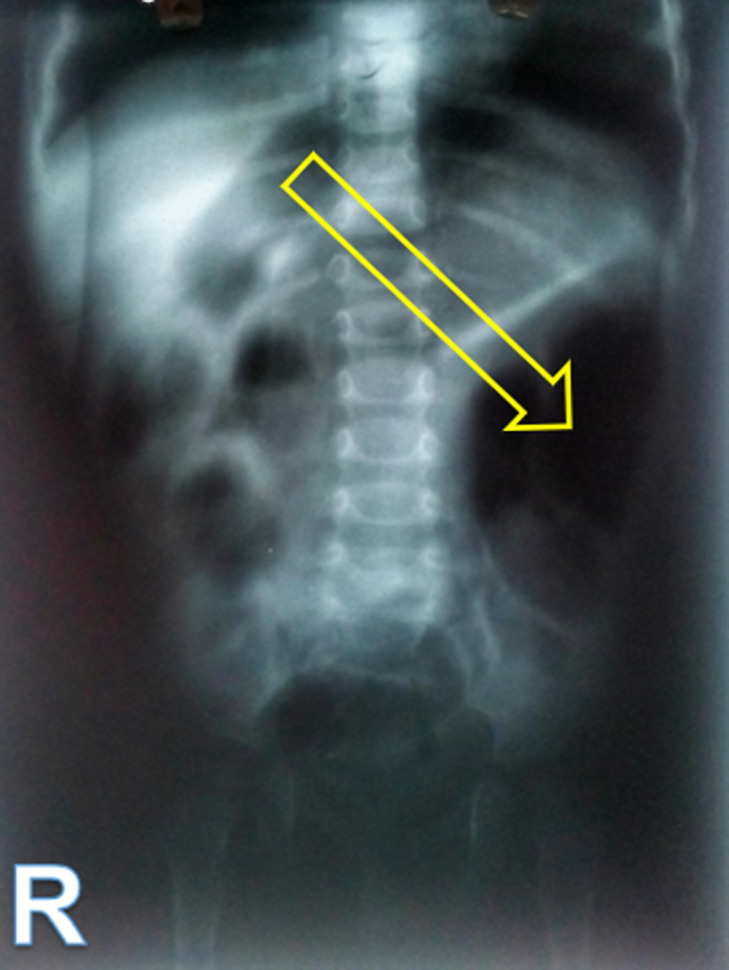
plain abdominal X-ray showing a distended intestinal loop without air-fluid level in the left lumbar region (yellow arrow)
